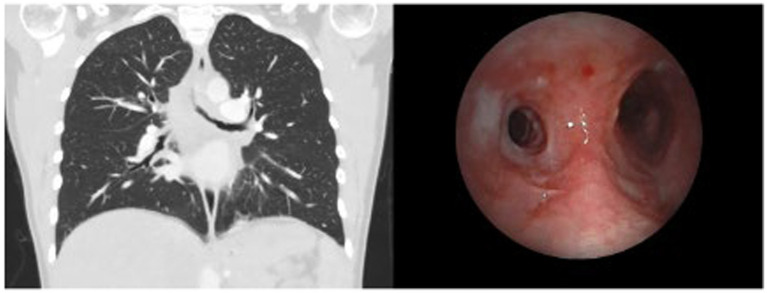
Case 2: Coronal CT scan (left) showing severe stenosis of the left main bronchus, and corresponding bronchoscopic view (right) demonstrating the endoluminal narrowing at the same anatomical site.
